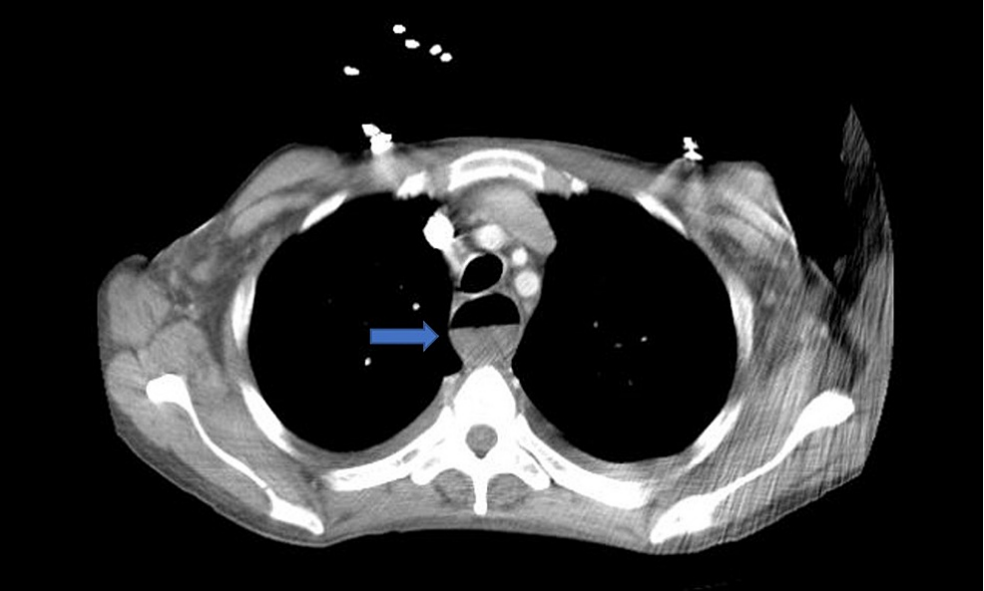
CT chest shows a distended esophagus to about 2.5-3 cm with an air-fluid level (blue arrow)
